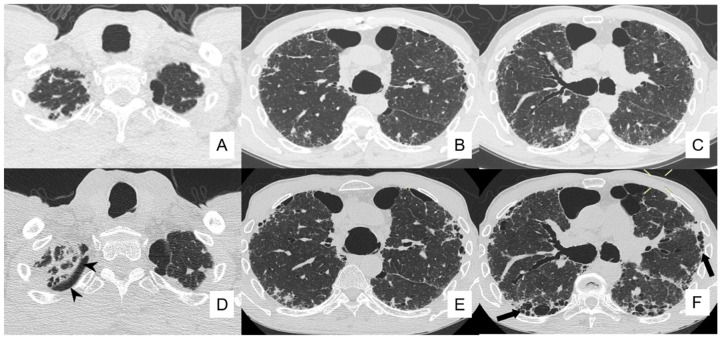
A 61-year-old man with IPF. Images (A–C) (acquired in 2016) demonstrate CT signs of interstitial disease (reticulations and bronchiectasis). Bullae of paraseptal emphysema in the bilateral apexes are also evident. HRCT images acquired in 2018 (D–F) identified a minimum flap of pneumothorax (7 mm in thickness, located in right apex).
